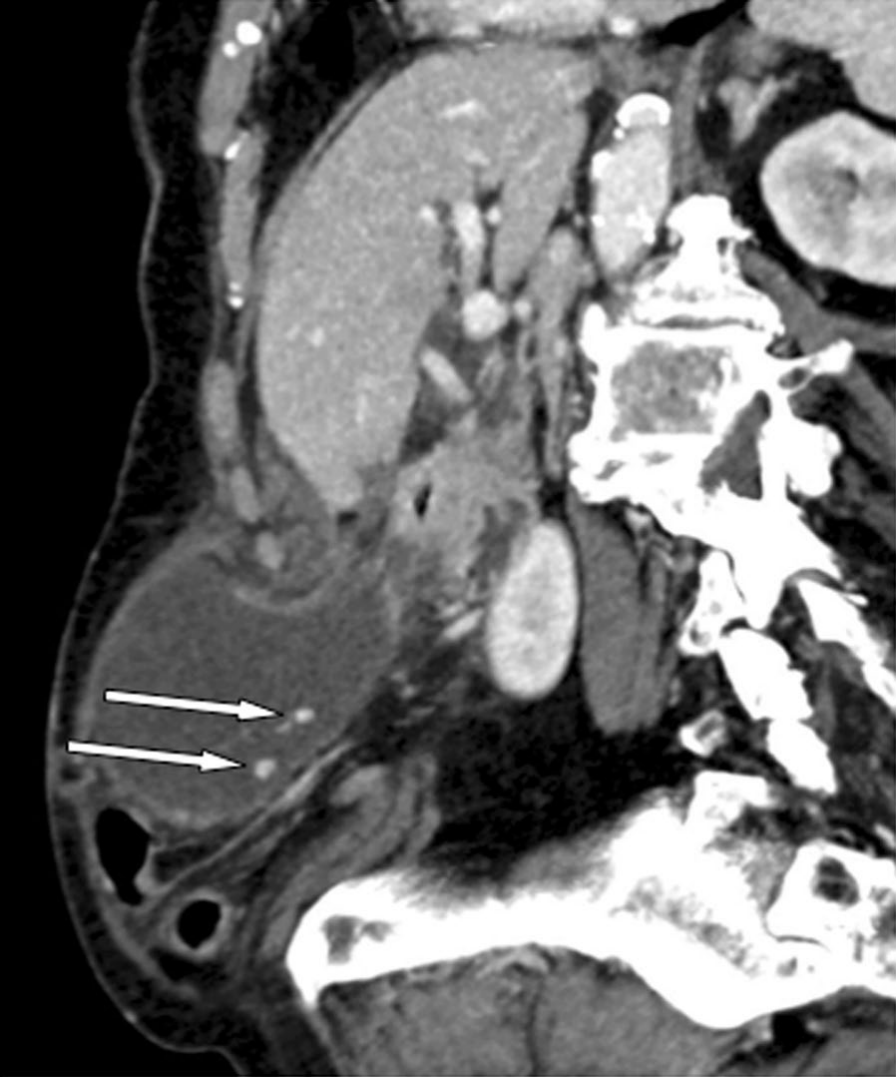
CT-scan pre-operative view. Note the absence of gallbladder fundus fixation, the presence of gallstones (*arrows*). On coronal planes we suspected a volvulus (*whirl-sign*).
